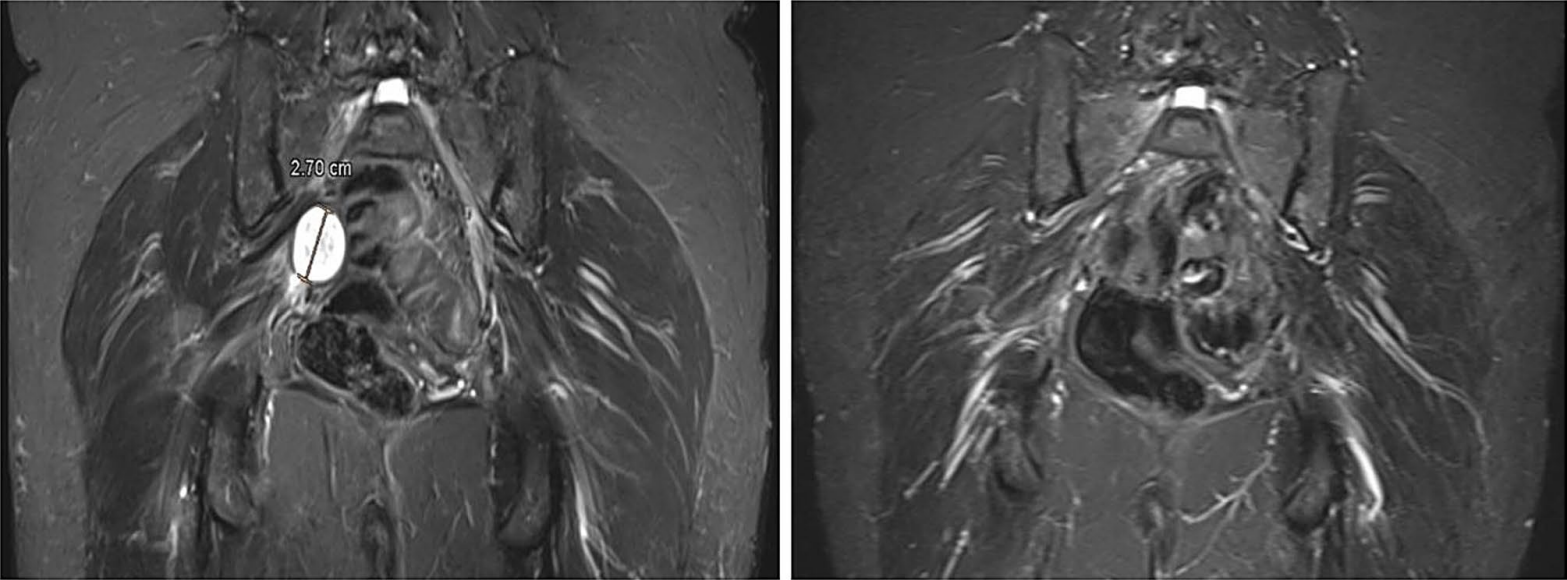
Pre- and first postoperative MRI after 3 months
